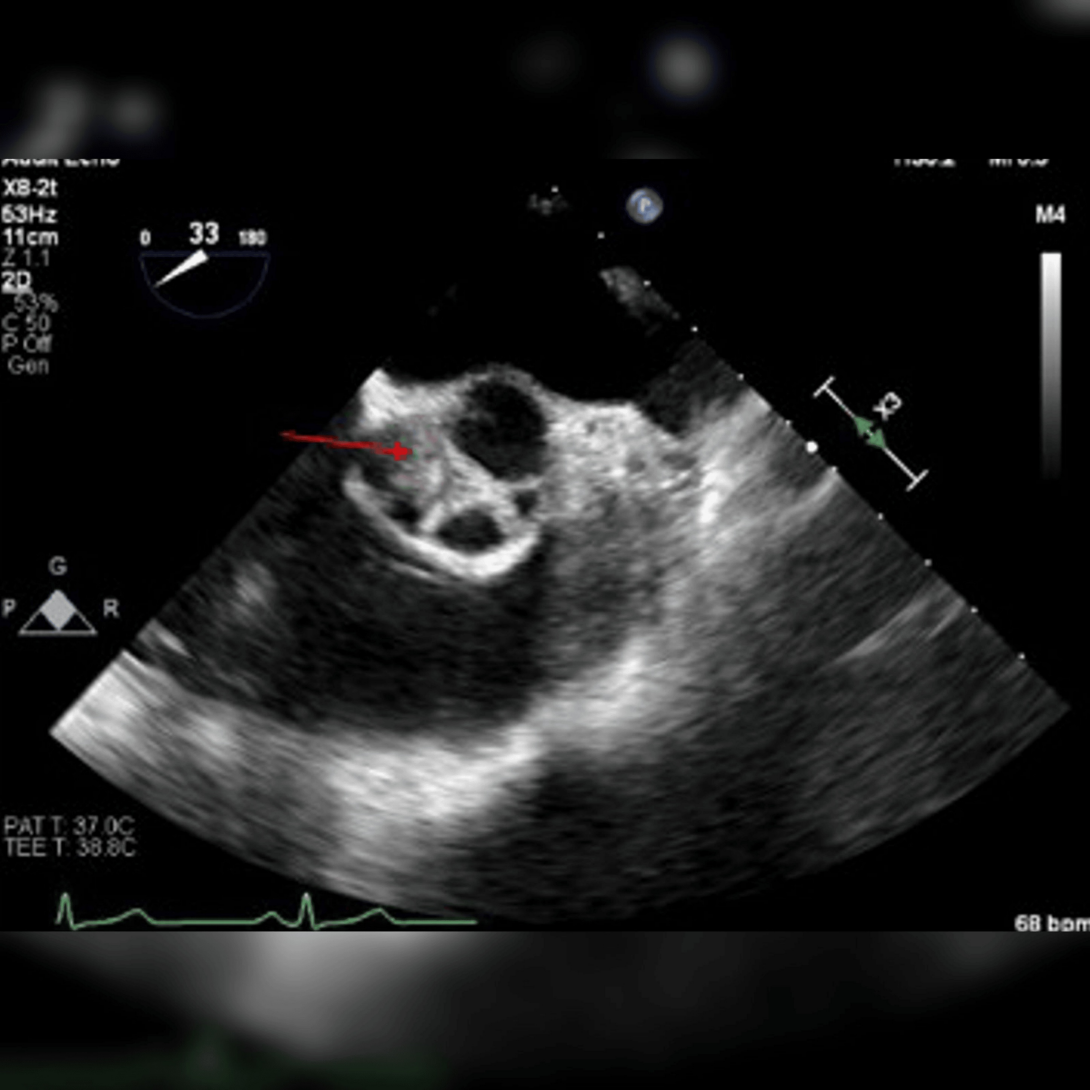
Transesophageal echocardiograph showing broad based fibroelastoma of the noncoronary cusp (red arrow)
